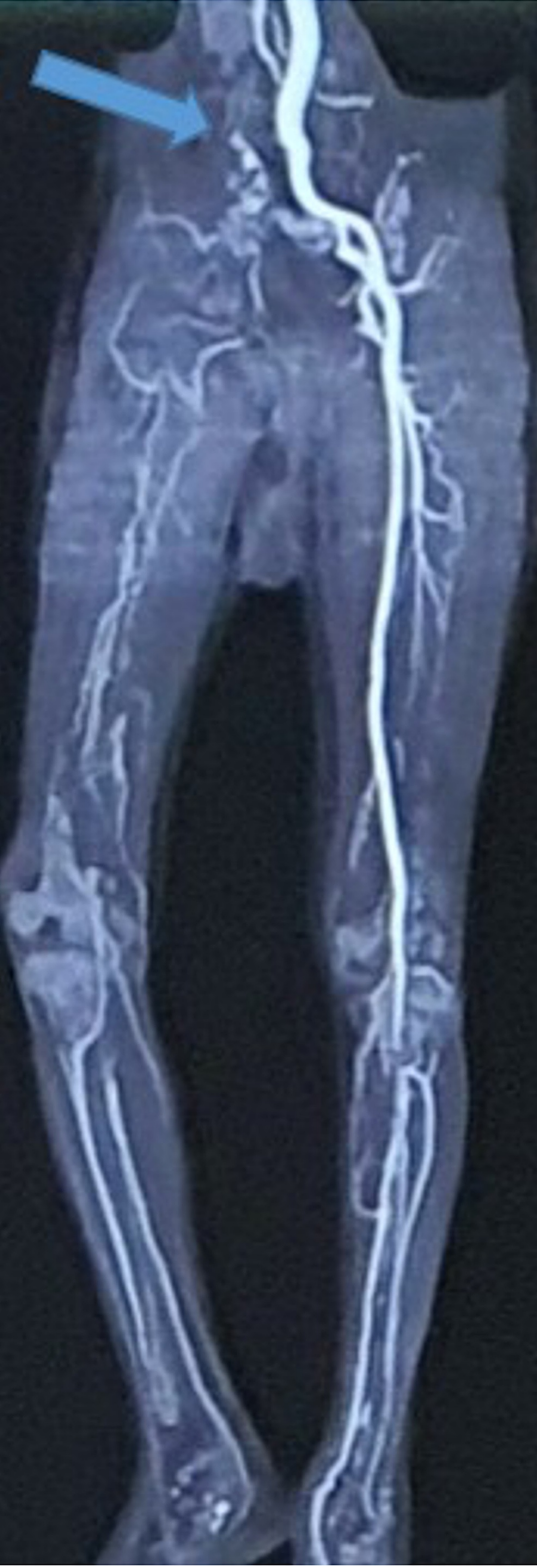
Computerized tomography angiography (CTA) (coronal view): The blue arrow indicates sudden abruption of contrast from right common femoral artery (CFA) and no contrast opacification in the popliteal artery (PA), anterior tibial artery (ATA), posterior tibial artery (PTA), and dorsalis pedis artery (DPA)
